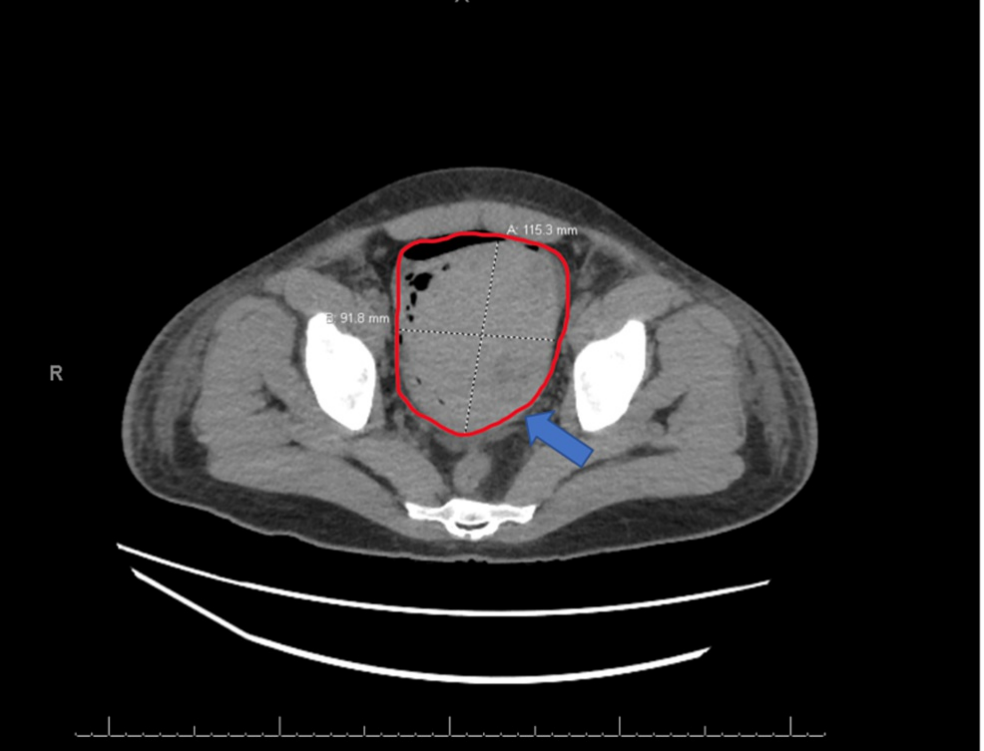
CT abdomen and pelvis with contrast: 12-cm hyperdense lesion in the urinary bladder, likely representing hematoma (blue arrow pointing at the hematoma circled in red). mm: Millimeters.
